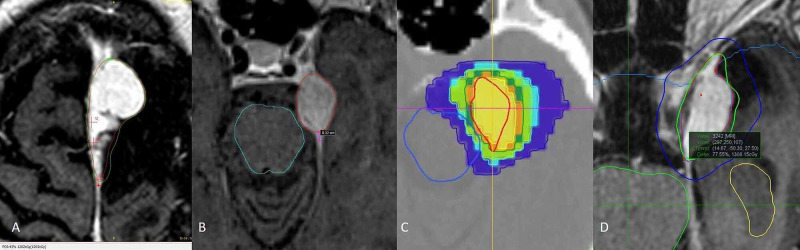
Plans performed with the different treatment systems CK, CyberKnife; Gad, gadolinium; MaxDis, maximum distance; MinDoseT, minimum dose at the dural tail; MPDis, midpoint distance; MPDose, midpoint dose; MRI, magnetic resonance imaging; Tomo, TomoTherapy. (A) Axial T1 Gad showing an interhemispheric meningioma treated with gamma; the green isodose line corresponds to the prescription dose of 14 Gy where an attempt to cover much of the tail is performed; at the most posterior aspect of the tumor there is a red mark signaling the end of the dural tail. The dose at this point can be read at the bottom of the image and corresponds 12.2 Gy at MinDoseT. (B) Contouring on MRI T1 Gad with Tomo of an anterior tentorium, left meningioma outlined in red, posterior to the tumor the measurement line in magenta signals the MPDis that is 3.2 mm. (C) Same meningioma in Tomo’s treatment planning station; prescription dose is 14 Gy, and the dose measurement at MPDose is 14.3 Gy. (D) Axial T1 Gad showing a treatment plan, isodose curves for CK of a left cavernous sinuous meningioma being prescribed 14 Gy represented with the green isodose line; most exterior curve in blue represents dose gradient; the outside measurement dot in the most posterior aspect of the lesion signals the MinDoseT receiving 13 Gy as can be read in the green box at the middle of the image.
